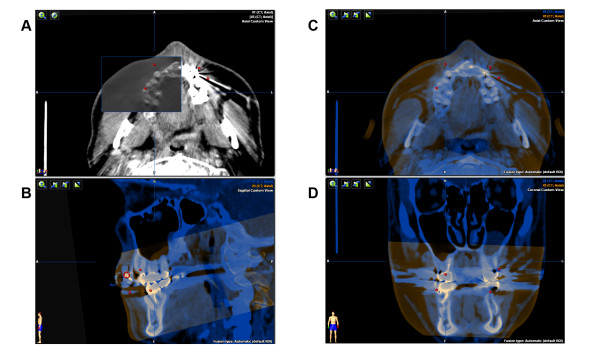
Superimposing of the subvolume. Superimposing of the subvolume with the initial CT scan (demonstrated in different planes A-D).
